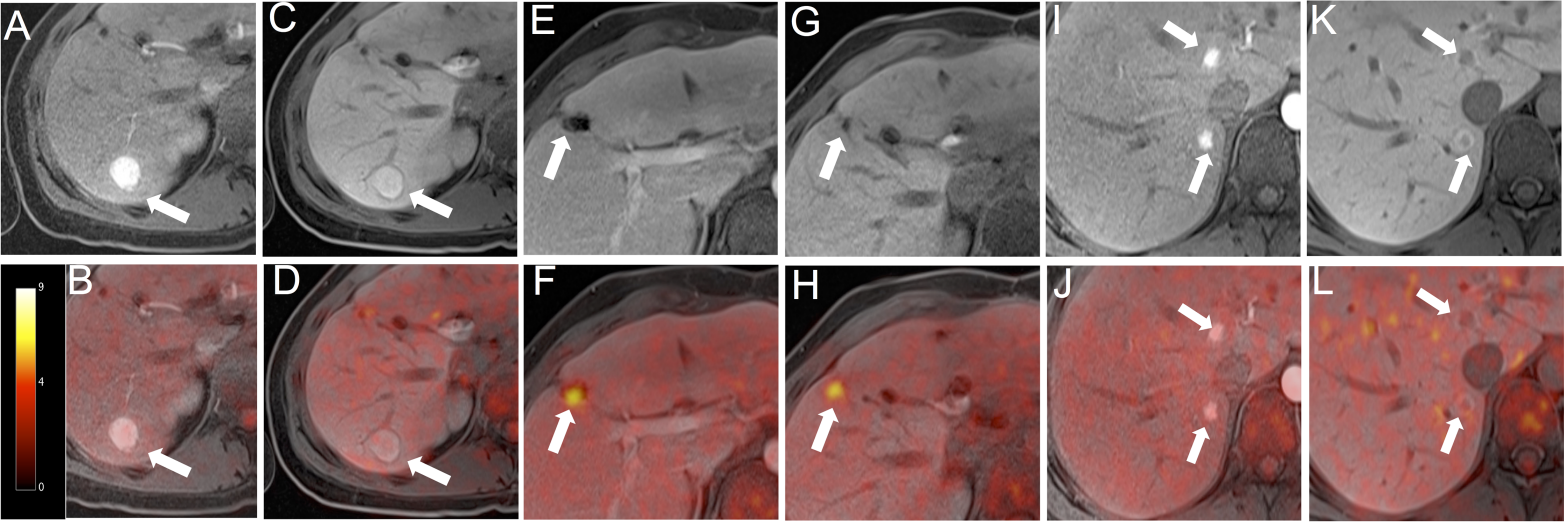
A 25-year-old female patient with a history of colorectal cancer presented multiple liver lesions after surgery. The FNH in the right liver shows an arterial contrast-agent enhancement (A) and is still hyperintense in the liver-specific contrast phase (C). No significant ^18^F-FDG-uptake is seen (B, D). A second lesion in the right liver is rated as a colorectal liver metastasis due to incomplete resection. Tumor lesion is neither detectable by MRI without liver-specific contrast phase nor with liver-specific contrast phase (E; G). In fused PET/MR images (F; H) the remaining tumor tissue lesion could clearly be identified. Additional lesions near the liver hilus are adenomas with strong arterial contrast-agent enhancement (I). In the liver-specific contrast phase lesions are hypointense (K). Similar to the FNH, no significant ^18^F-FDG-uptake is seen (J, L).
